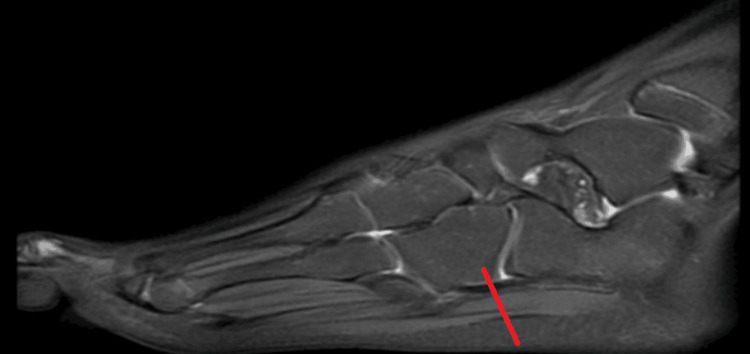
Control MRI Right Foot Control MRI shows complete resolution of bone marrow edema.
